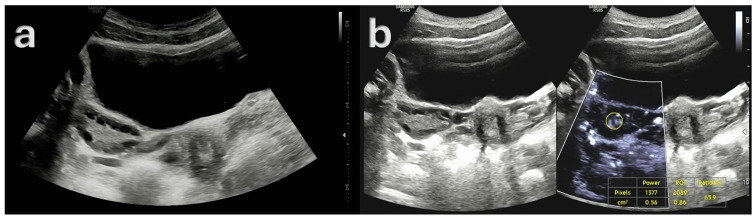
Ultrasound findings in a 23-year-old female patient with Type A polycystic ovary syndrome (PCOS) phenotype. (a) Gray-scale ultrasound image demonstrating polycystic ovarian morphology (PCOM), with multiple small peripheral follicles and a prominent central stroma. (b) MV-Flow Doppler ultrasound image showing increased vascularity within the ovarian stroma, with a high vascularity index (VI), consistent with elevated stromal blood flow (ratio: 65.9%). These imaging features, in conjunction with clinical and biochemical evidence of hyperandrogenism and ovulatory dysfunction, are characteristic of the classic Type A PCOS phenotype.
